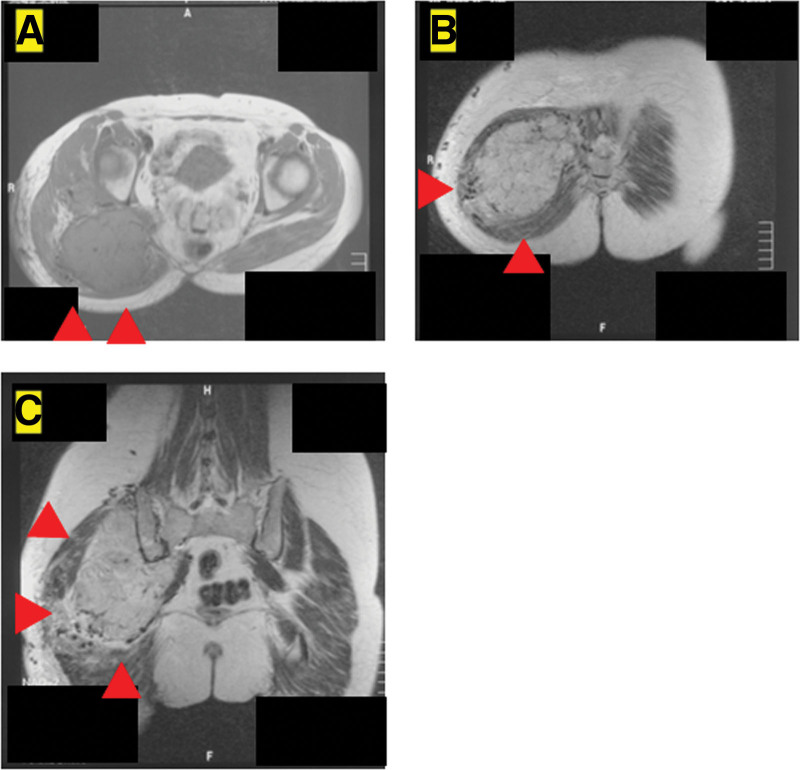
Axial T1-weighted (A) and T2-weighted (B) magnetic resonance imaging (MRI) of the buttocks. Coronal T2-weighted image (C) of the buttock. The red arrowheads indicate the tumor mass.
